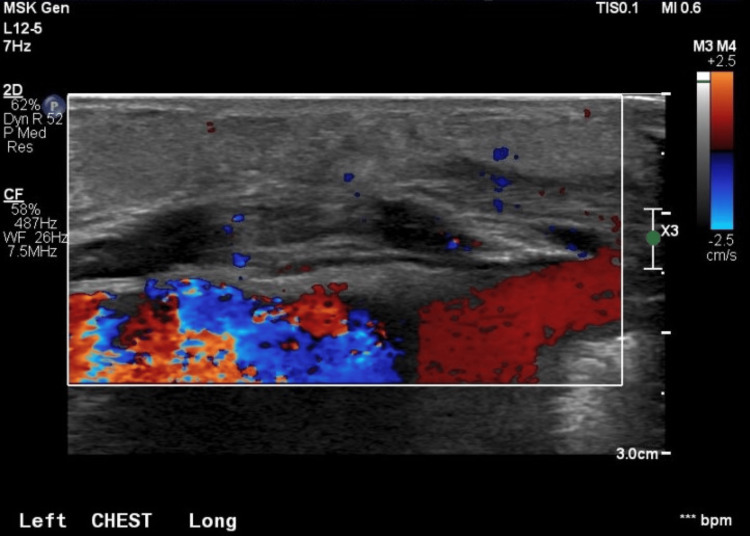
Ultrasound imaging showing a lack of vascular component increase in subcutaneous tissue
